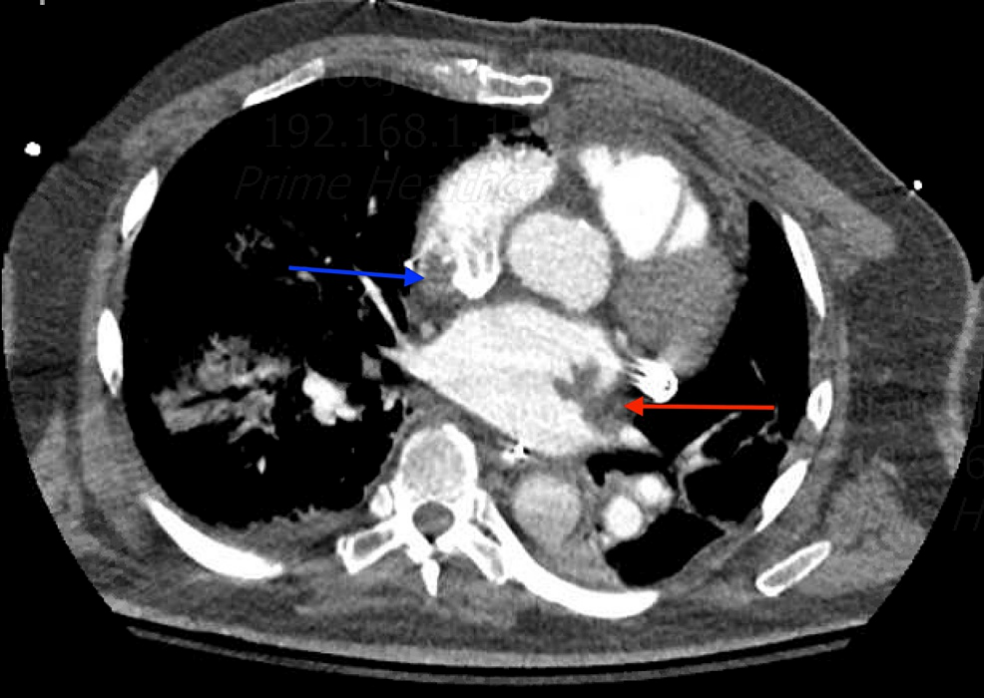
CTA of the chest showing extensive thrombosis in the left atrium (red arrow) and the right atrium (blue arrow) CTA: computerized tomographic angiogram
